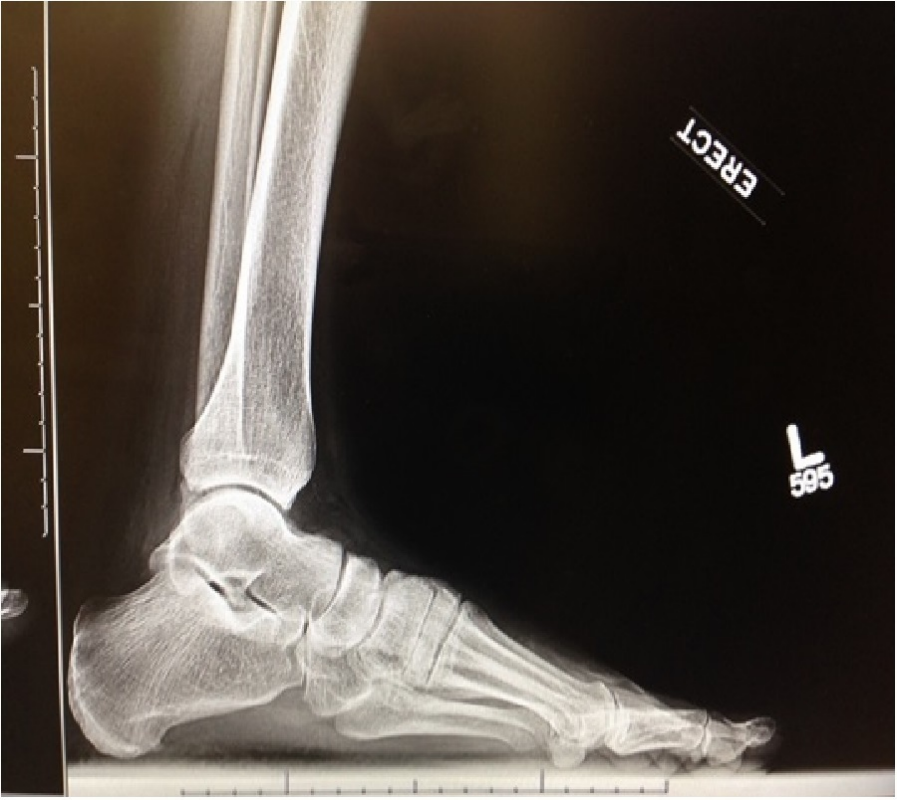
Preoperative weight bearing lateral radiograph of the left foot demonstrating reduction of the first metatarsal cuneiform joint despite dorsal capsular disruption and instability of the joint
